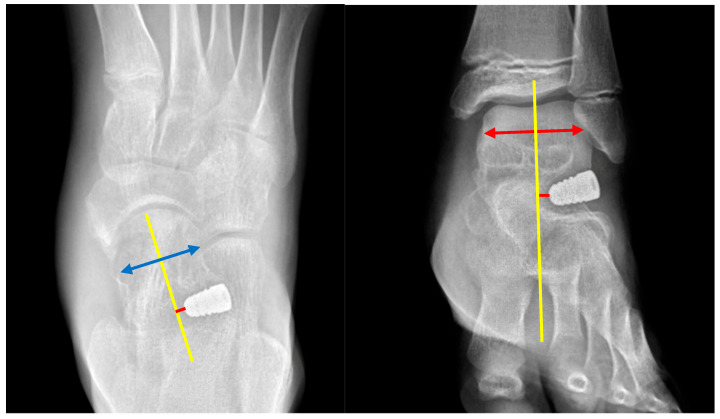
An assessment of implant positioning on anteroposterior radiographs. The yellow line represents the longitudinal axis of the talus. The double-headed blue arrow indicates the width of the talar neck in the foot AP view, and the double-headed red arrow indicates the width of the talar body in the ankle AP view. The red line indicates the perpendicular distance from the implant tip to the talar axis.
